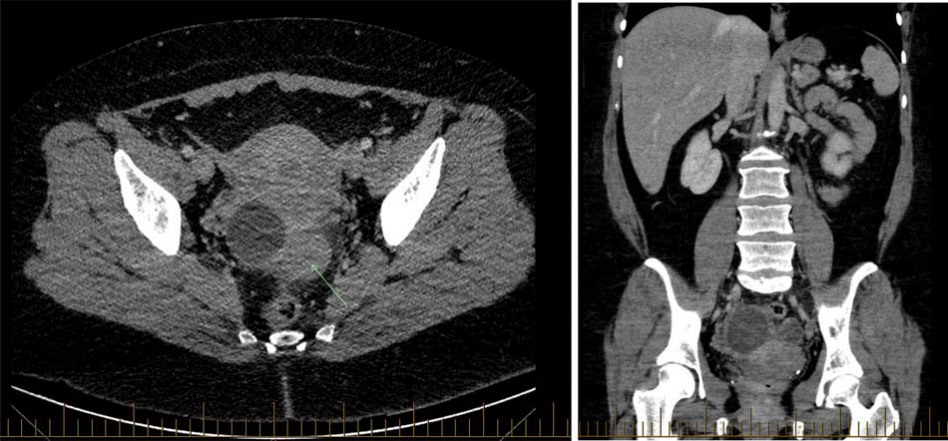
CT abdomen demonstrating pelvic pathology.
